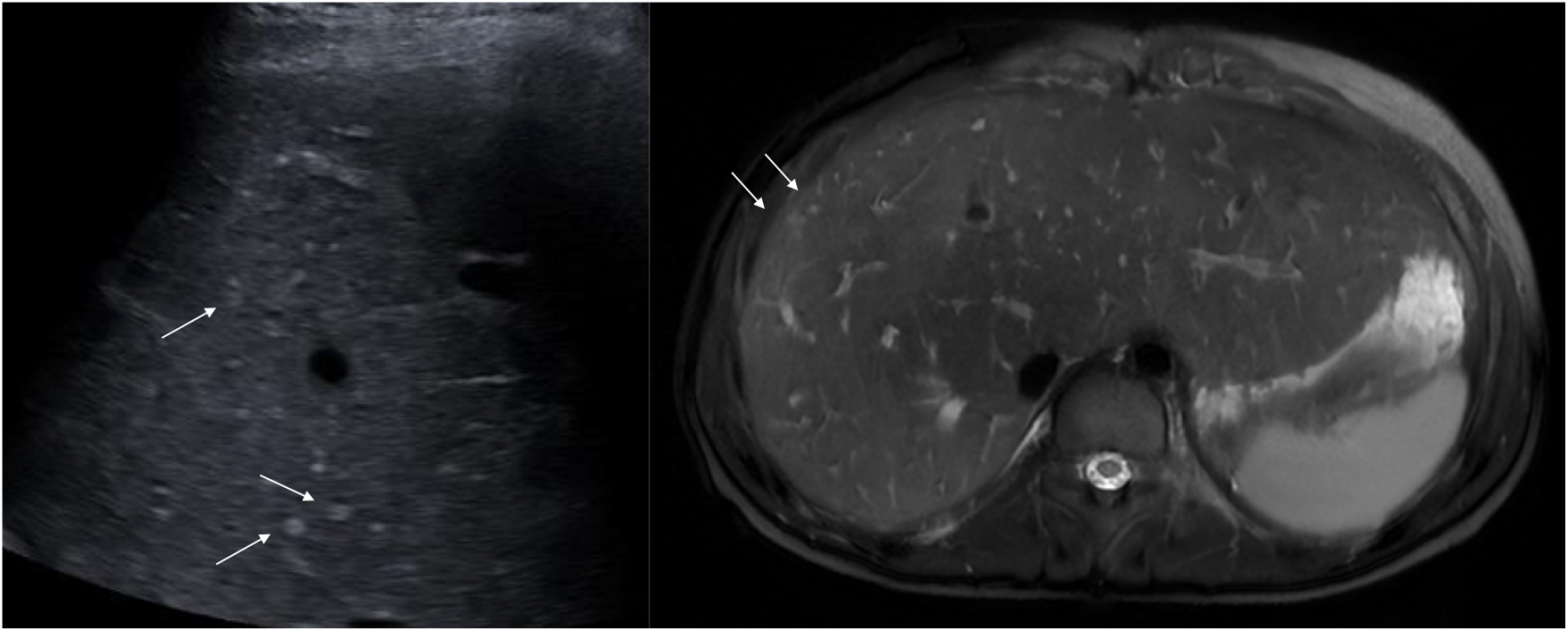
Typical hyperechogenic vascular nodules 3–5 mm (arrows) on ultrasound (left) and corresponding nodules with high signal on axial T2-weighted MRI (right). Note the generally increased T2 signal in the parenchyma as an expression of congestion (right). MRI, magnetic resonance imaging
